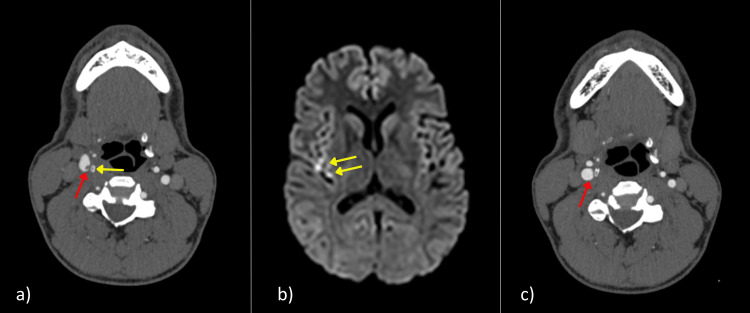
Imaging features of right internal carotid artery dissection in a 37-year-old male following a Brazilian Jiu-Jitsu chokehold. a) CT scan of the neck at presentation demonstrates an acute dissection flap of the medial wall of the right internal carotid artery (red arrow) immediately adjacent to the superior tip of the right greater cornu of the hyoid bone (yellow arrow). b) MRI diffusion-weighted imaging (DWI) sequence demonstrates a small area of acute ischemia infarction involving the right posterior insular cortex (yellow arrows). c) CT scan of the neck performed eight weeks after presentation shows remodeling of the small intimal dissection flap with resolution and return to normal appearance (red arrow).
